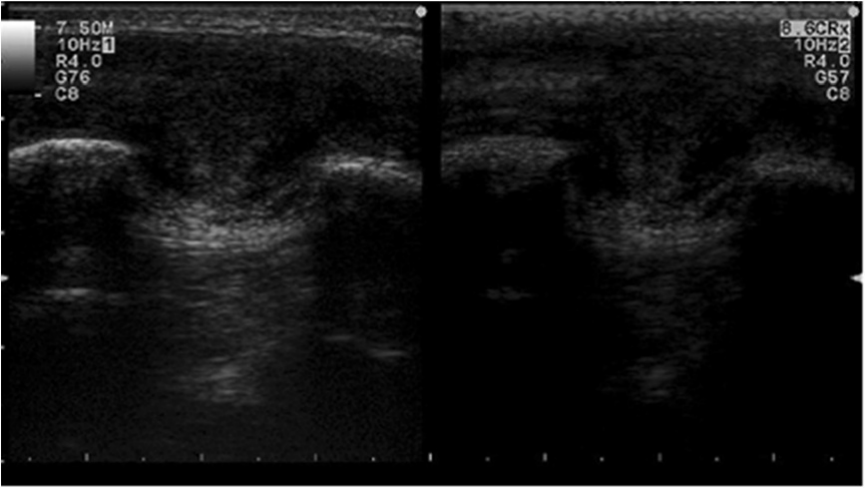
Postoperative contrast-enhanced ultrasonographic image. Double image that shows the results after radiofrequency ablation of the desmoid tumour under contrast-enhanced ultrasonographic guidance (SonoVue; Bracco Imaging, Milan, Italy). The absence of contrast enhancement demonstrates the successful treatment.
